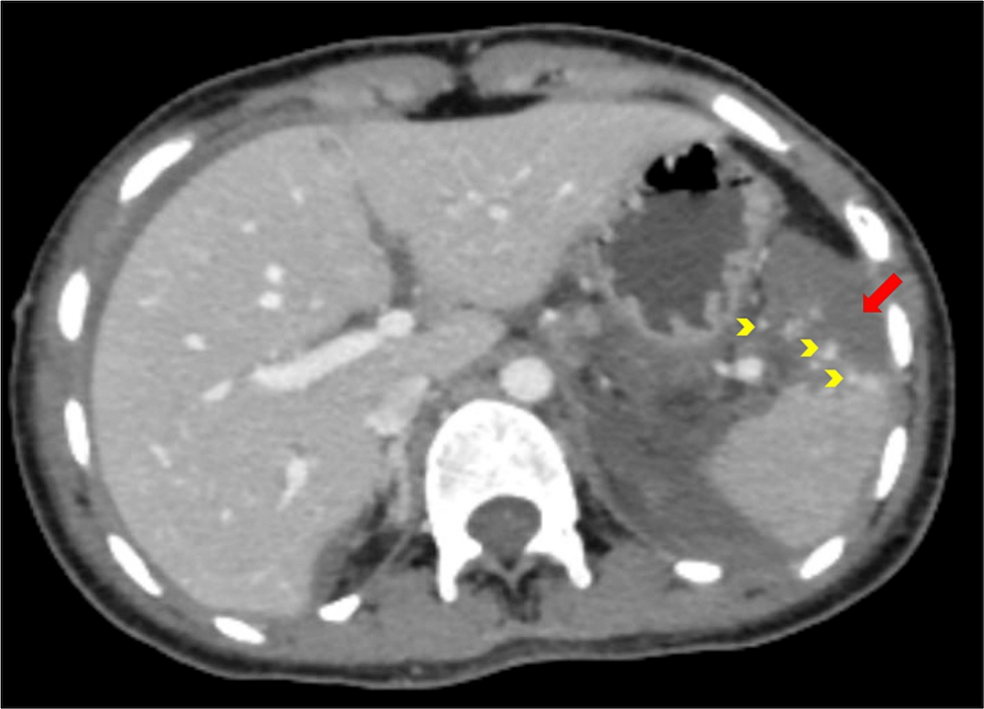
AAST grade IV splenic injury Contrast-enhanced CT of the abdomen in the portal venous phase shows areas of devascularisation involving >25% splenic parenchyma (red arrow) along with focal hyperdense traumatic pseudoaneurysms (yellow arrowheads) in the interpolar region and lower pole, as well as subcapsular and perisplenic hematoma. These findings are suggestive of AAST grade IV splenic injury. AAST: American Association for the Surgery of Trauma
